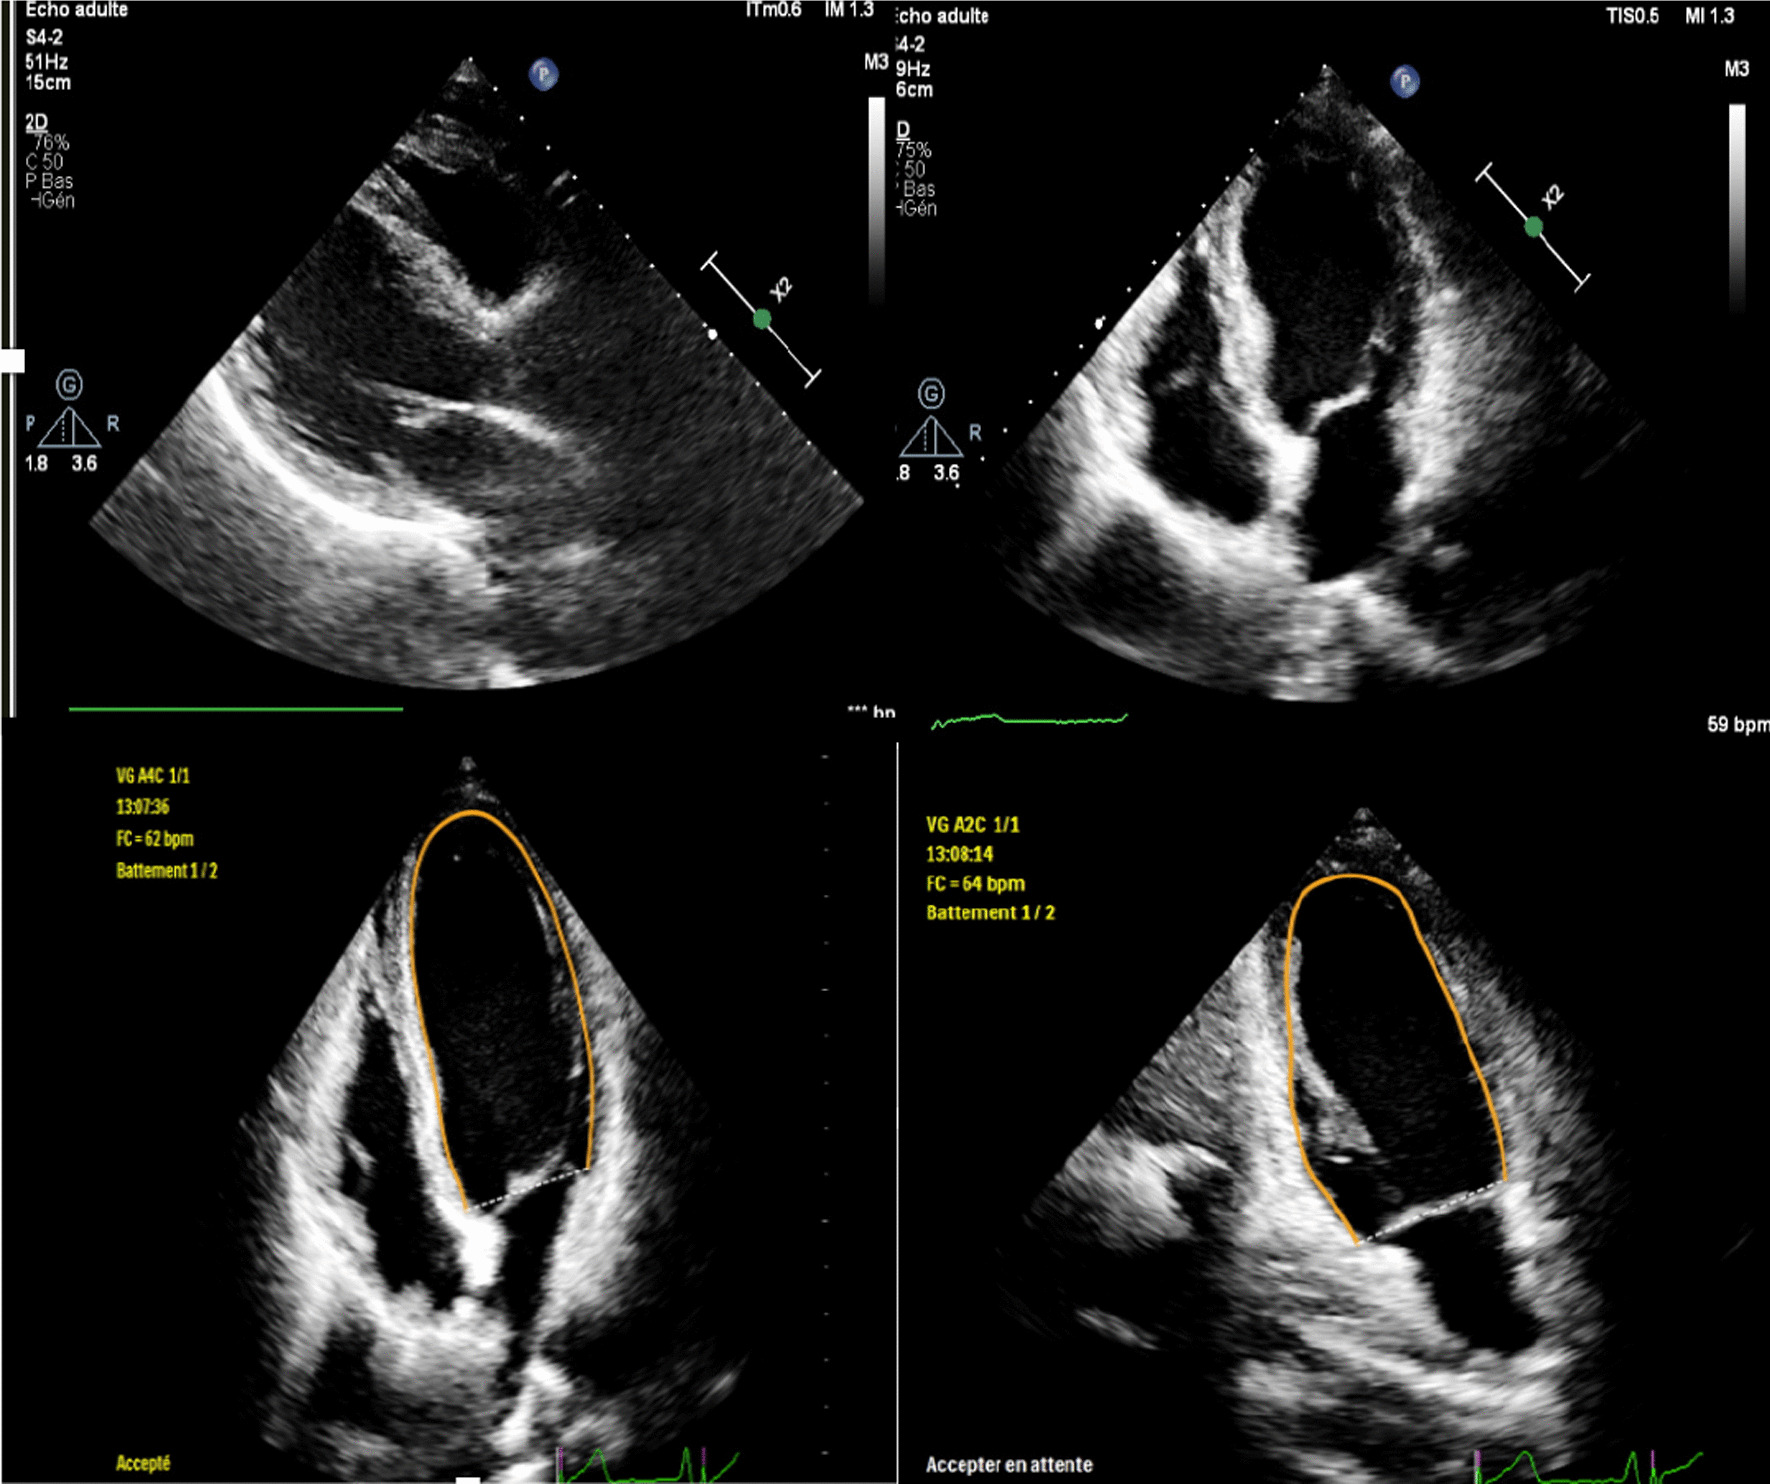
Initial transthoracic echocardiogram findings: ischemic cardiopathy aspect with moderate ventricular dysfunction. No pericardial effusion was noticed
